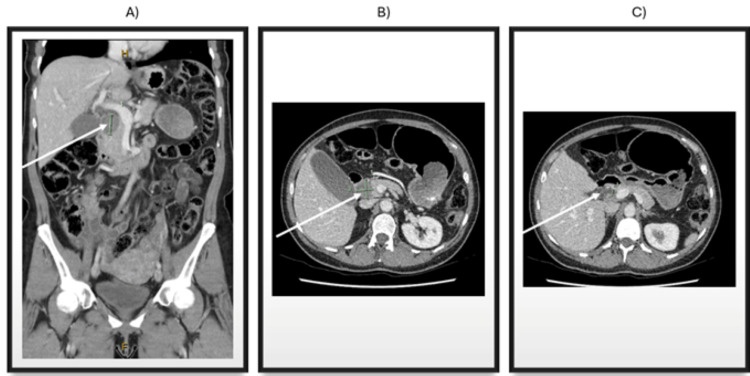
Abdominal portal venous contrast CT of cystic pancreatic lesion (white arrow) in A) coronal section demonstrating 20.5 mm tall, B) axial section demonstrating 26.2 mm long and 28.9 mm wide, with C) CBD dilated to 10 mm. CT: Computed tomography, CBD: Common bile duct
